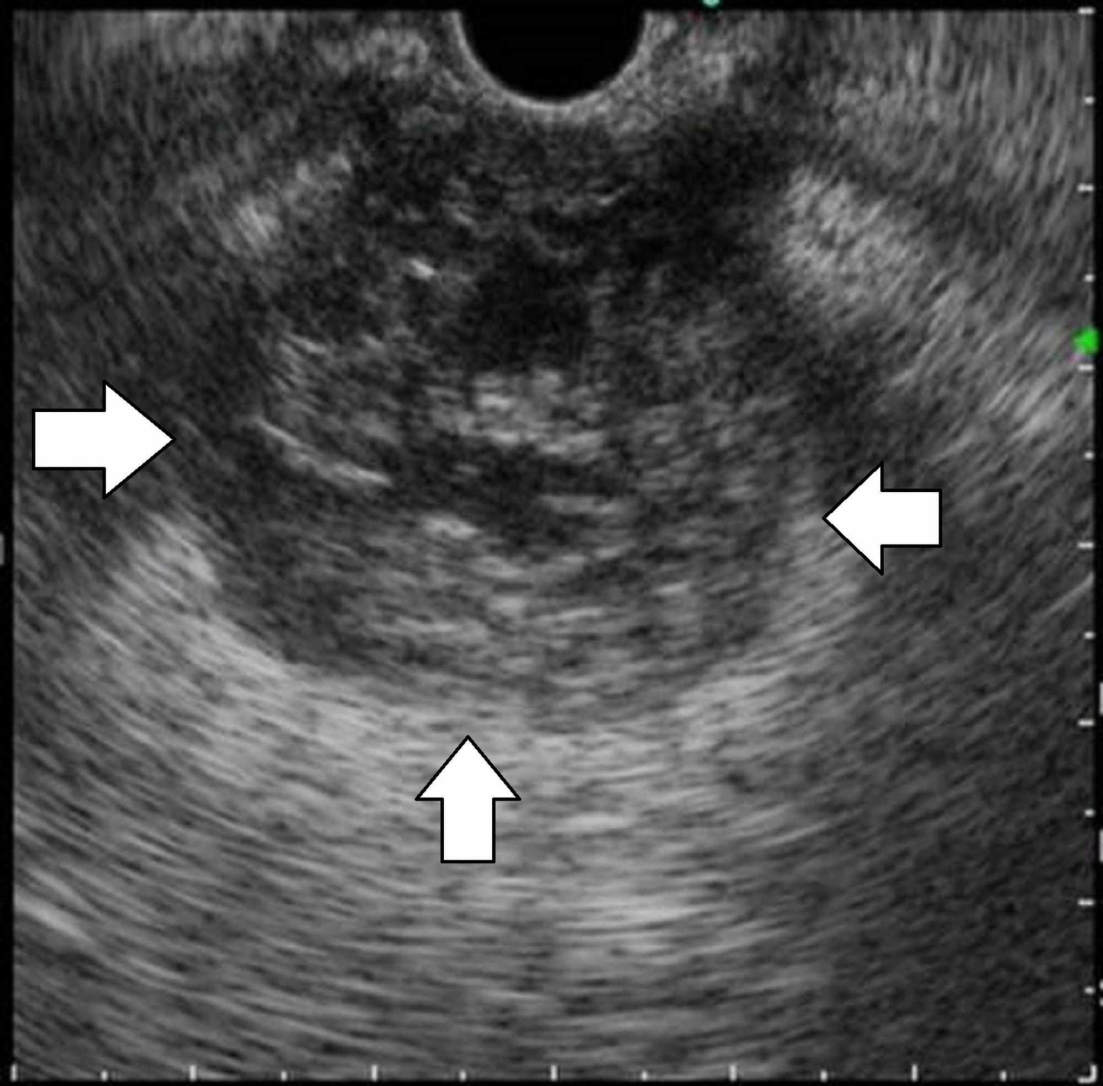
Endoscopic ultrasonography The internal echo of the tumor revealed a mosaic pattern in endoscopic ultrasonography findings.
